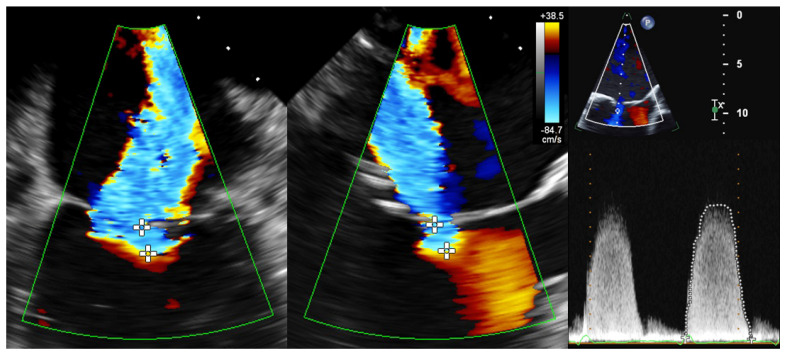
Determination of 2D PISA in biplane 2D color Doppler dataset and CW Doppler dataset. Echocardiographic images with 2 orthogonal slice planes of the 2D color Doppler dataset (mid-systolic) with Nyquist velocity of 38.5 cm/s (left); determination of PISA radius in 2 planes (diameters with crosses), MR-VTI (dotted outline), and MR-Vmax in the CW Doppler dataset (right) for calculation of regurgitation area. Abbreviations: 2D = two-dimensional; PISA = proximal isovelocity surface area; CW = continuous wave; MR-VTI = mitral regurgitation velocity–time integral; MR-Vmax = maximum mitral regurgitation velocity.
